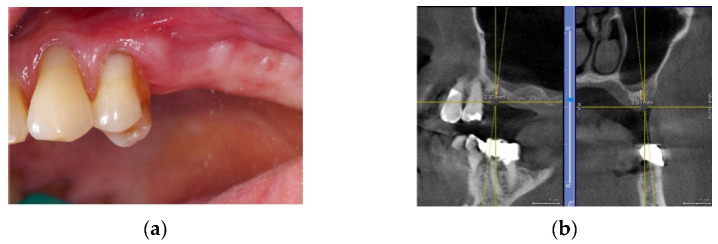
(a,b): First quadrant clinical aspect and TAC X-ray image.
